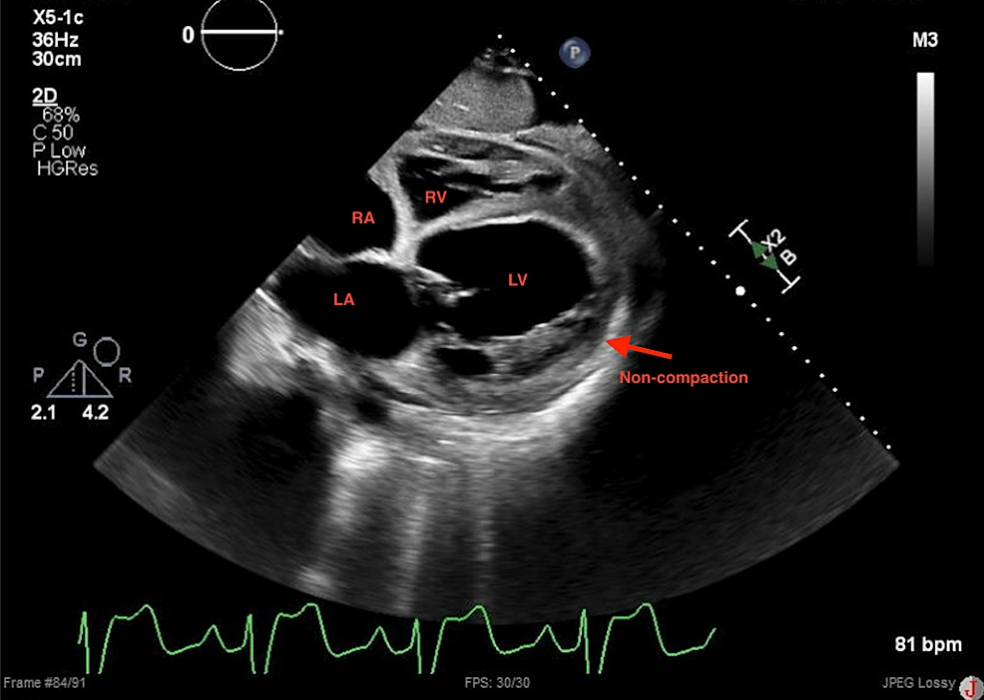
2D transthoracic echocardiogram (subcostal view) showing a dilated left ventricle and signs of LVNC LVNC: Left ventricular non-compaction
